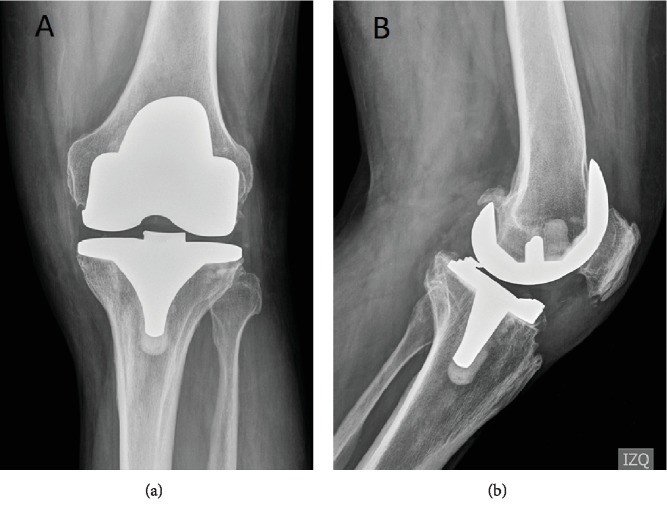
Radiograph seven months after left knee arthroplasty: (a) anteroposterior left knee view and (b) lateral left knee view. No signs of loosening or osteolysis were found.
